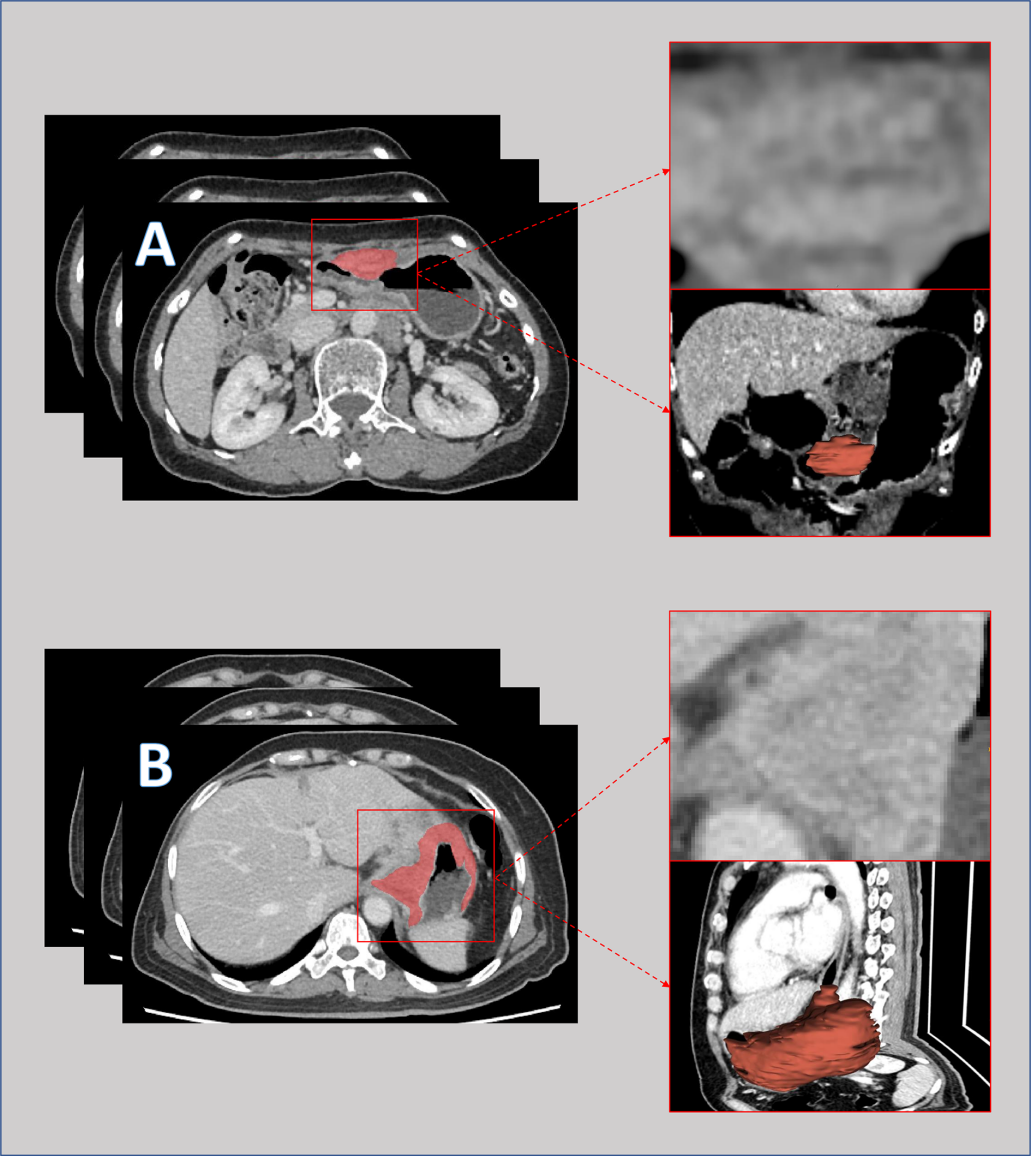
Pre-intervention venous-phase computed tomography images of a patient with major response (A) and a patient with minor response (B) to neoadjuvant chemotherapy. The lesions were delineated slice by slice and merged into a 3-dimensional region for features extraction.
